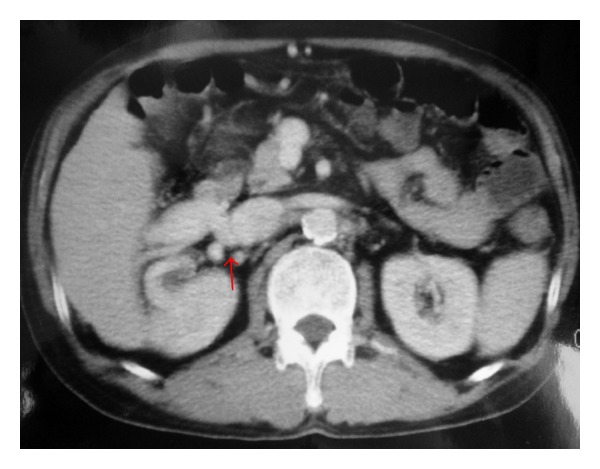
Computed tomographic findings. The portosystemic shunt (arrow) can be seen above the right kidney.
